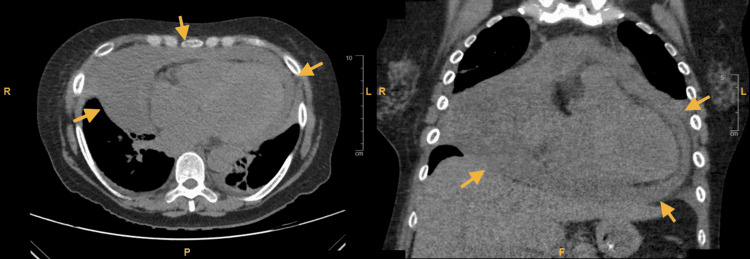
Chest CT. Large hemopericardium (arrows) CT, computed tomography.
